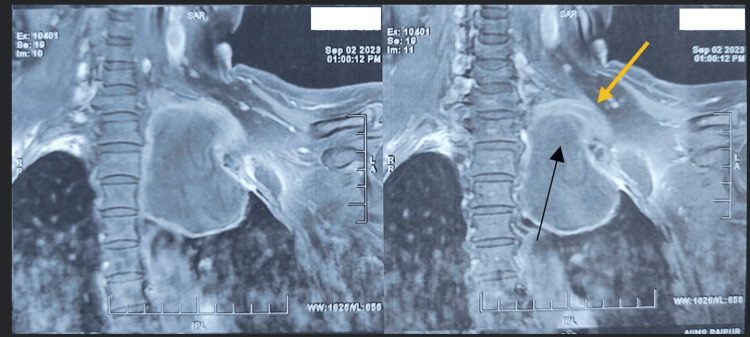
An MRI of the brachial plexus demonstrating the cyst (pointed with the black arrow) abutting the left brachial plexus (pointed with the yellow arrow).
